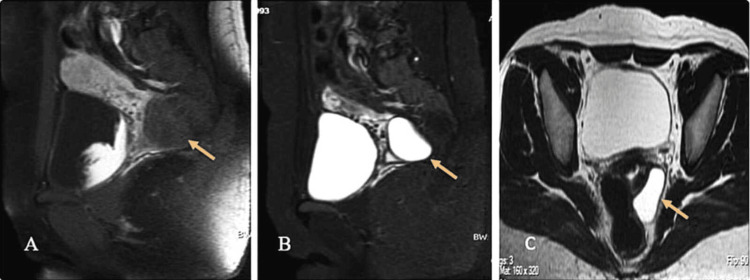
MRI of the pelvis (A) Sagittal T1-weighted and (B) sagittal STIR images demonstrating a well-defined cystic lesion (orange arrows) in the left posterolateral rectovaginal pouch, extending to the posterior lip of the cervix, appearing hypointense on T1-weighted and hyperintense on STIR images. (C) Axial T2-weighted image demonstrating a hyperintense cystic lesion in the left posterolateral rectovaginal pouch (orange arrow). STIR, short tau inversion recovery
